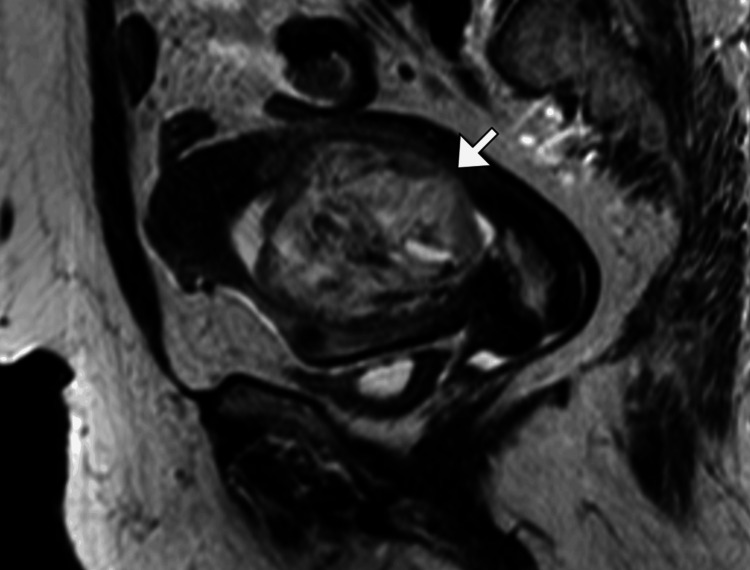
Sagittal T2-weighted MRI of the pelvis Sagittal MRI demonstrates a large endometrial mass (arrow) distending the uterine cavity with heterogeneous signal intensity and preserved endometrial–myometrial junction, consistent with papillary serous endometrial carcinoma.
